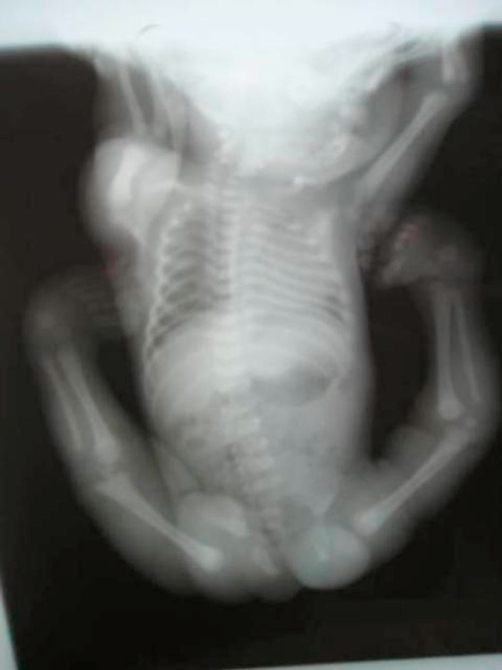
Anteroposterior whole skeleton radiograph at the age of one month showed multiple contractures associated with clubfoot, bilateral dislocation of elbows, hips and knees (most characteristically, anterior dislocation of the tibia on the femur), and short metacarpals with cylindrical fingers lacking the usual tapering were the prime skeletal features associated with undermineralization and overtubulation of the long bones were present.
